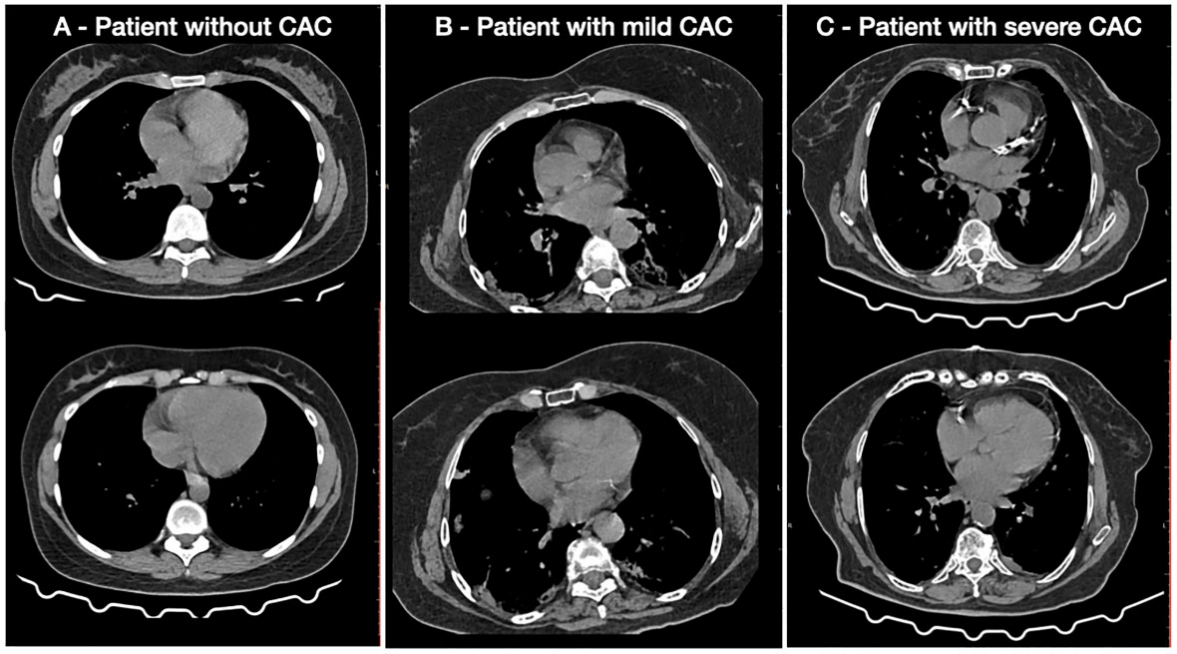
Coronary calcification in chest computed tomography scans. A, Examples of a patient without coronary artery calcification (CAC), B, a patient with mild CAC (presence of calcification in two coronary segments), and C, a patient with severe CAC (presence of calcification in six coronary segments).
